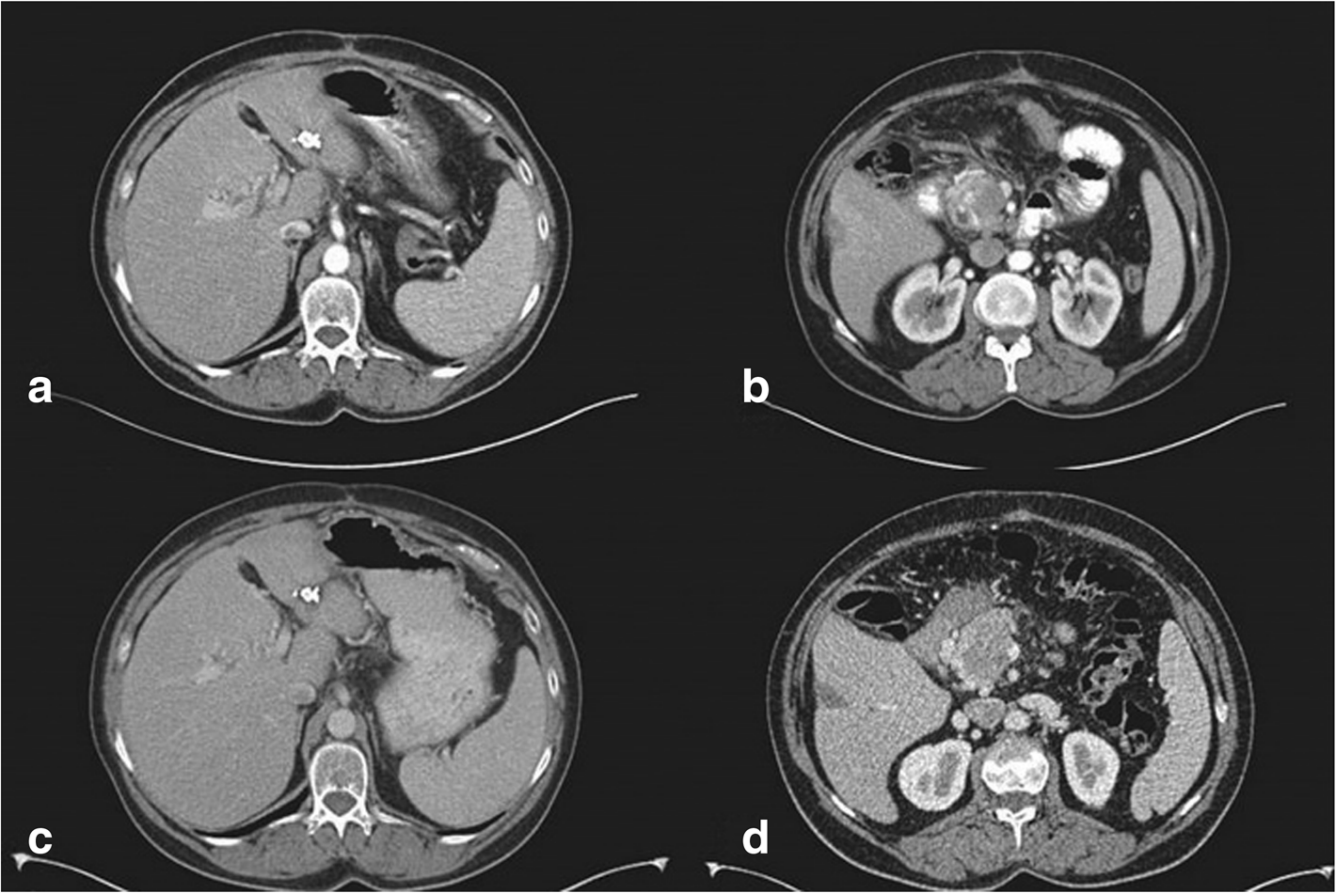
Follow-up CT scan. a Site of segment III resection. No new liver lesions detected 6 months after the operation. b Pancreatic head lesion after IRE with no obvious changes or progression of disease 6 months after the operation. c Twelve months after the operation, the liver remains free of new lesions. d Twelve months after the operation, the pancreatic mass shows no progression or enlargement
